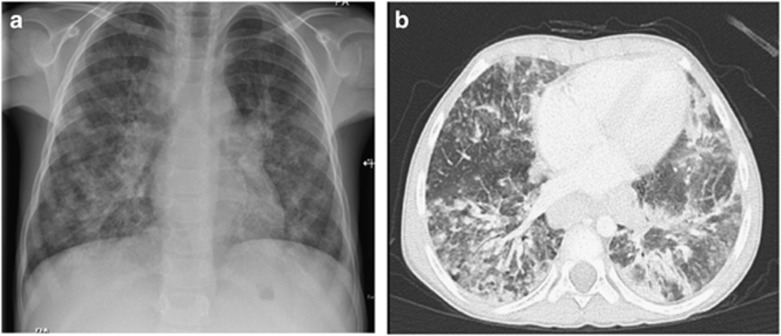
Radiological findings. Chest X-ray and high-resolution computed tomography scan showing interstitial lung infiltrates and hilar lymphadenopathy.
